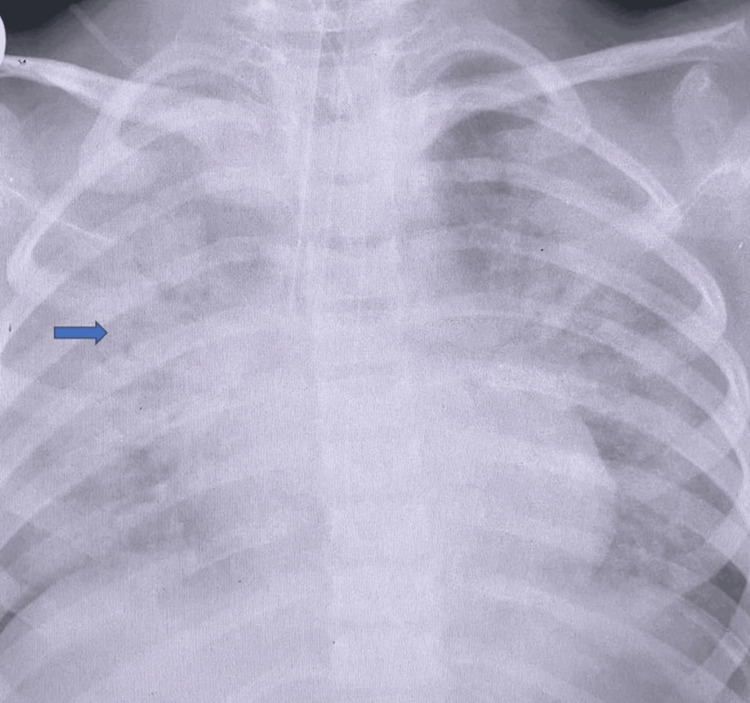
Chest X-ray showing acute respiratory distress syndrome. Chest X-ray showing acute respiratory distress syndrome after three days of admission upon admission to the intensive care unit, as demonstrated by the blue arrow.
